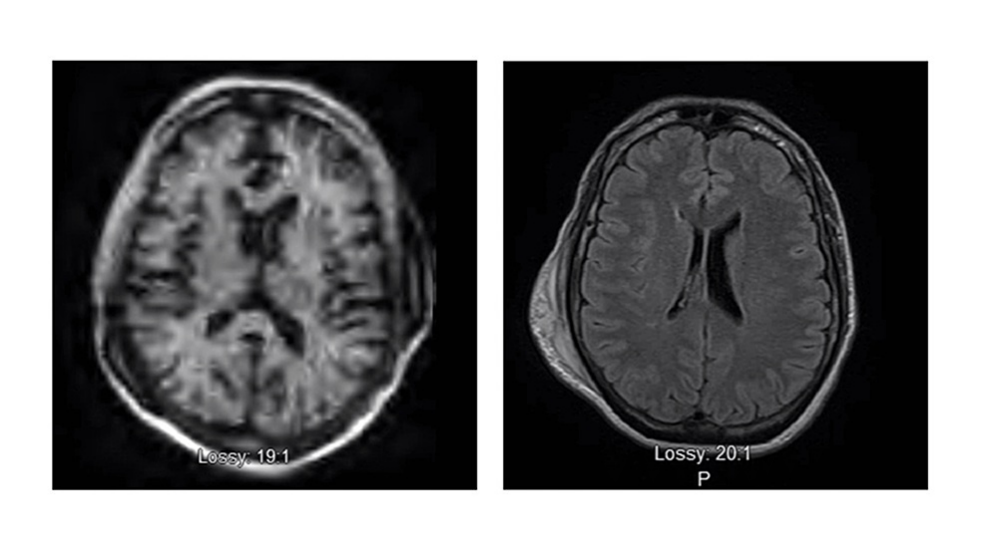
T1 axial MRI. Left - pMRI; right - traditional T1 MRI of the same patient. MRI, magnetic resonance imaging; pMRI, portable magnetic resonance imaging
